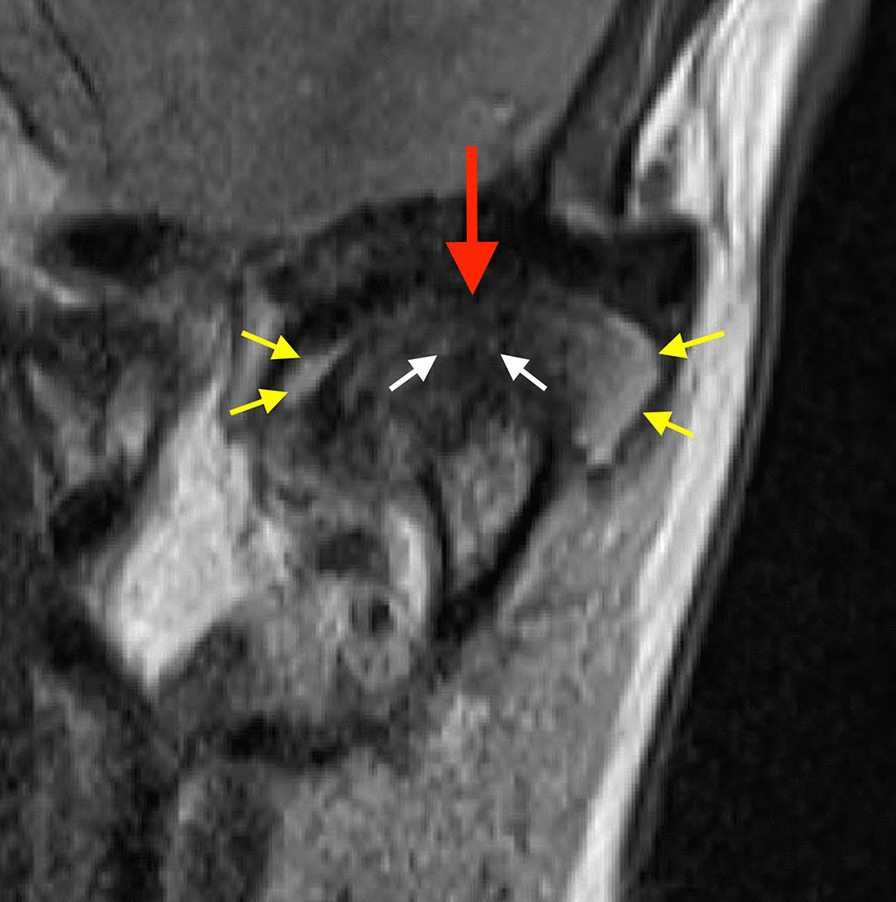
Closed–mouth-related MR images in a 61-year-old female with a 18-month history of left TMJ pain, a TMJ pain-side-related clinical diagnosis of TMJ AR, and the MRI finding of disk displacement without reduction, CE, bone marrow edema, and effusion. Coronal MR image shows condyle with CE (red arrow), bone marrow edema (white arrows), and effusion in the superior joint compartment (yellow errors)
